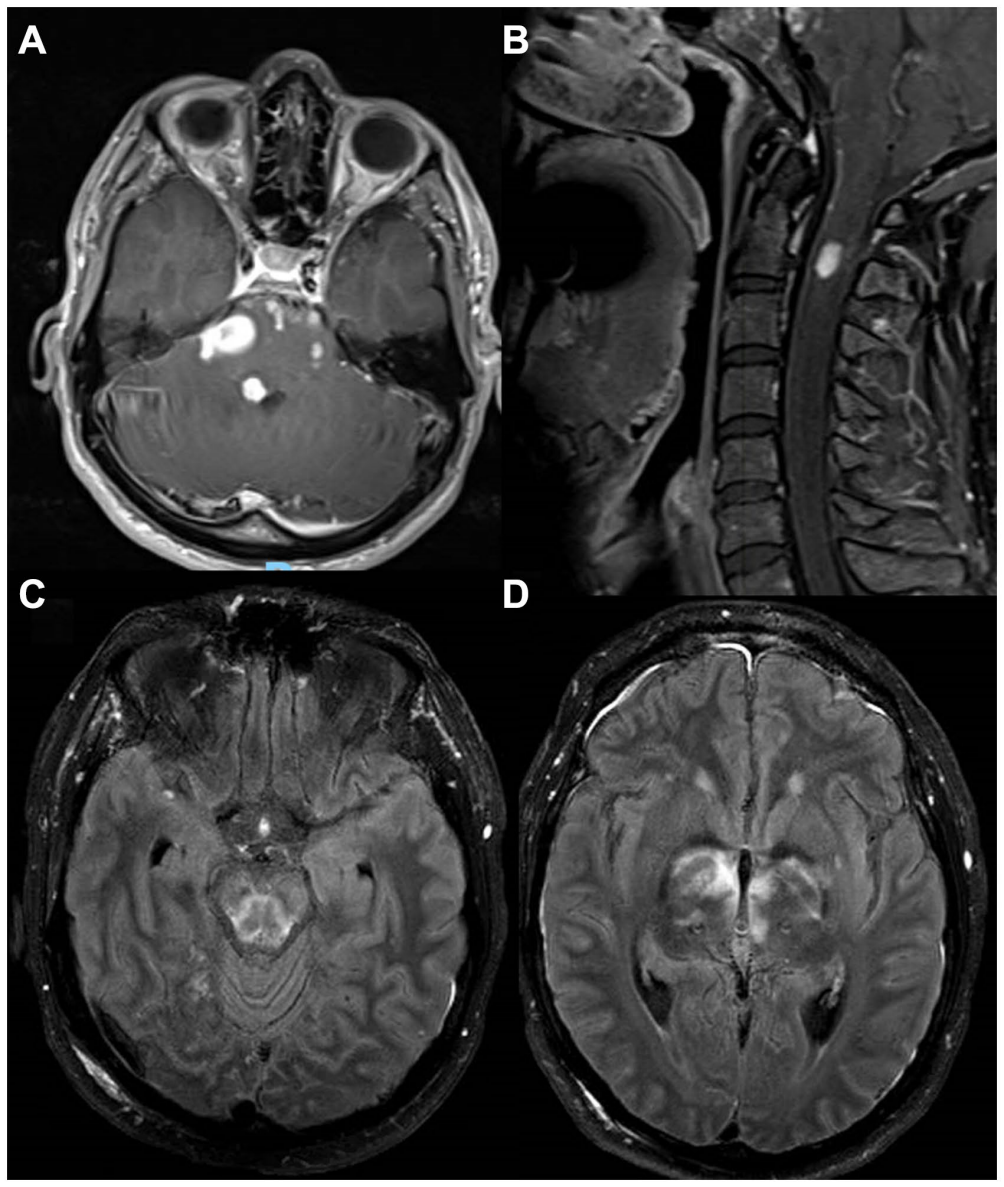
MRI images from a patient with NEMO deficiency and *Mycobacterium haemophilum* infection. A 28-year-old man with NEMO deficiency and *Mycobacterium haemophilum* infection with multiple enhancing lesions and associated edema on postcontrast T1 images from the brainstem (A) and upper cervical cord (B). Despite treatment, he had persistent rhomboencephalitis on postcontrast T2-FLAIR 5 months later (C,D), eventually leading to his death. FLAIR, fluid-attenuated inversion recovery; NEMO, Nuclear factor-kappa B Essential Modulator.
